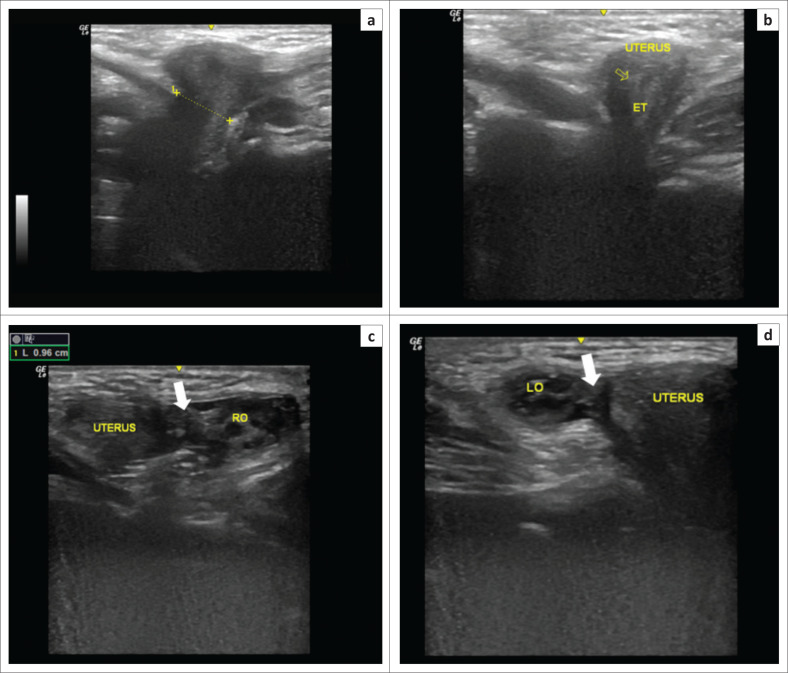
Ultrasound images of the left inguinal region (a to d) show a 9.6 mm size defect (dotted line in [a]) with the uterus herniating through it (void yellow arrow in[b]). The endometrial cavity (ET) can be seen within the uterus (b). Figure c and Figure d demonstrate the herniated right (RO) and left ovaries (LO) along with fallopian tubes (white solid arrows).
